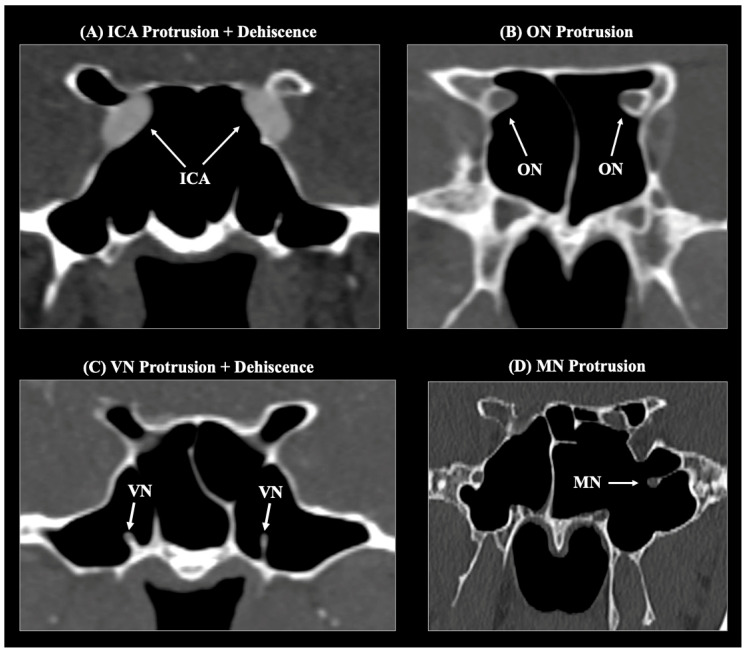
Representative computed tomography (CT) images demonstrating protrusion and dehiscence of key neurovascular structures into the sphenoid sinus (SS). (A) Internal carotid artery (ICA): bilateral protrusion with bony dehiscence. (B) Optic nerve (ON): bilateral protrusion into the superolateral wall of the SS. (C) Vidian nerve (VN): bilateral protrusion and dehiscence within a pneumatized SS showing combined lateral extension. (D) Maxillary nerve (MN): protrusion along the lateral wall of a full-lateral pneumatized SS. White arrows indicate the corresponding neurovascular structures.
